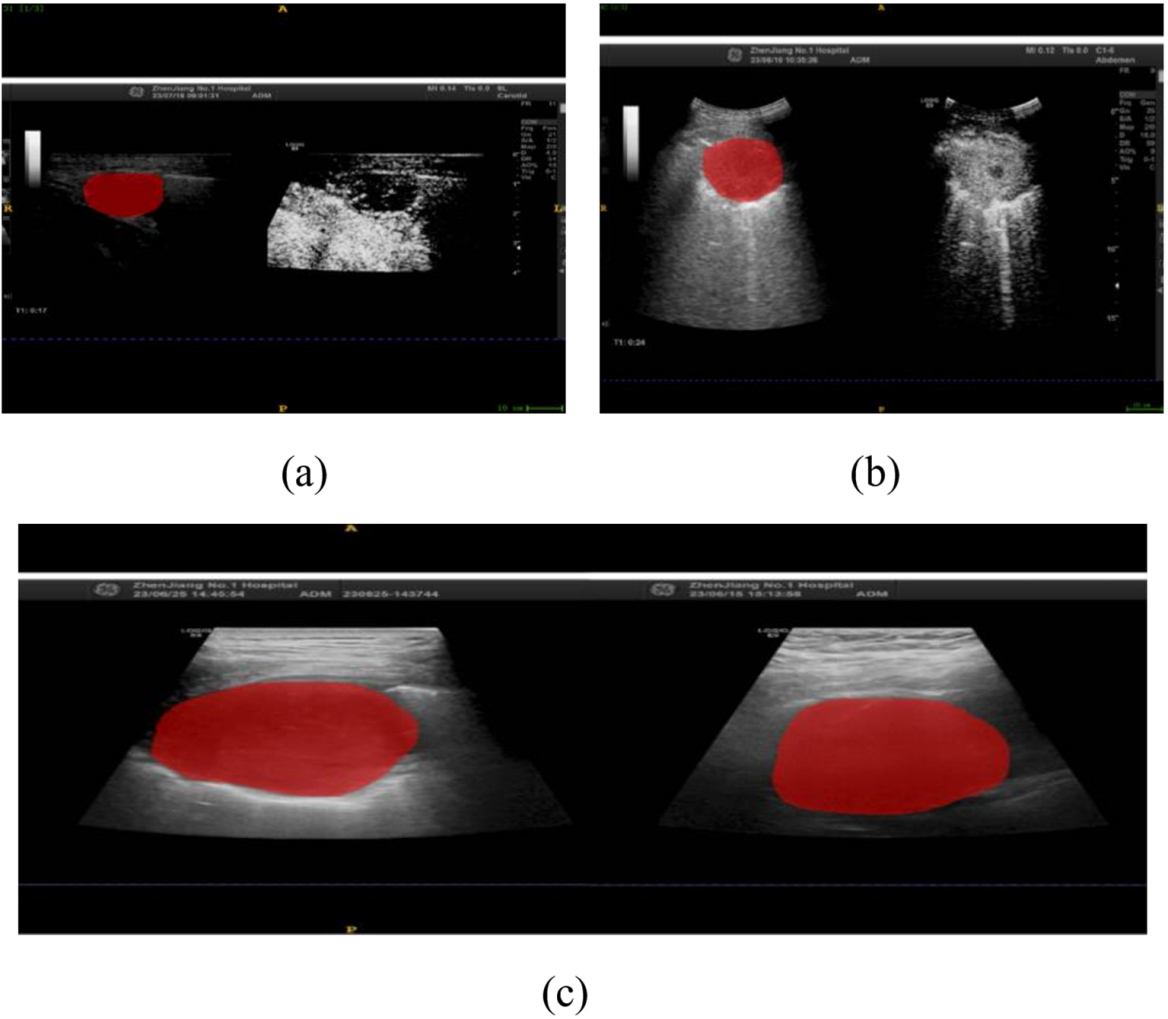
Ultrasound-Guided Puncture of Pulmonary Nodules (a) A 67-year-old male patient with left upper peripheral lung adenocarcinoma. (b) A 67-year-old male patient with left lower lung central squamous cell carcinoma. The red areas indicate the regions of interest (ROIs) for the punctures. (c) Gray-scale ultrasound images of the ROIs for both patients.
